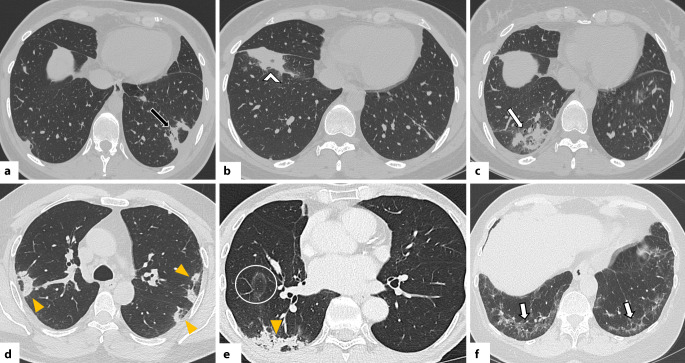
Axiale Aufnahmen der hochauflösenden Computertomographie (CT) zeigen für eine organisierende Pneumonie (OP) typische Befunde. a Periphere Konsolidierung im linken Unterlappen mit perilobulärem Muster (*schwarzer Pfeil*), vereinbar mit einer OP. b–c Verlaufskontrollen demonstrieren die typische Entwicklung der OP: migratorisches Verhalten und Wiederauftreten in anderen Arealen (*weißer Pfeilkopf*, *weißer Pfeil*). d, e Periphere Konsolidierungen mit perilobulärem Muster (*gelbe Pfeilköpfe*) sowie ein „reverse-halo sign“ (sog. „Atoll sign“, *weißer Kreis*). f OP-Muster mit Fibrose bei einem Patienten mit einem Antisynthetase-Syndrom: beidseitige lineare Verdichtungen in perilobulärer Verteilung (*weiße Pfeile*)
